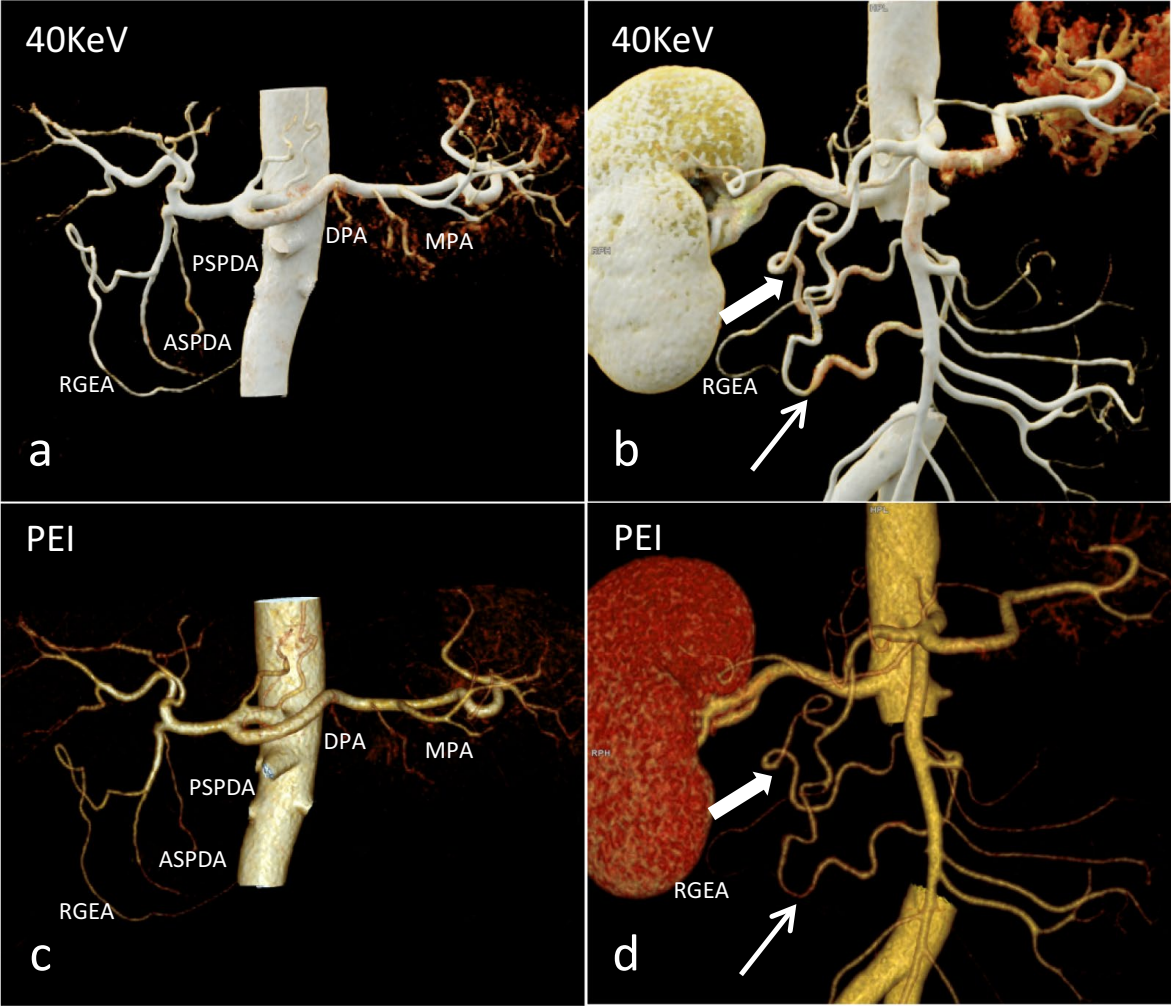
The upper (a, b) and lower (c, d) rows show the 40-keV MEI (+) and PEI images, respectively. a Volume Rendering Technology (VRT) of contrast-enhanced CT angiography shows the anterior superior pancreaticoduodenal artery (ASPDA), posterior superior pancreaticoduodenal artery (PSPDA), dorsal pancreatic artery (DPA) and magnificent pancreatic artery (MPA). b VRT shows standard anterior (thin arrow) and posterior (thick arrow) pancreaticoduodenal arcade. The visualization is better on the 40-keV MEI (+), compared to the PEI. RGEA = Right gastroepiploic artery
